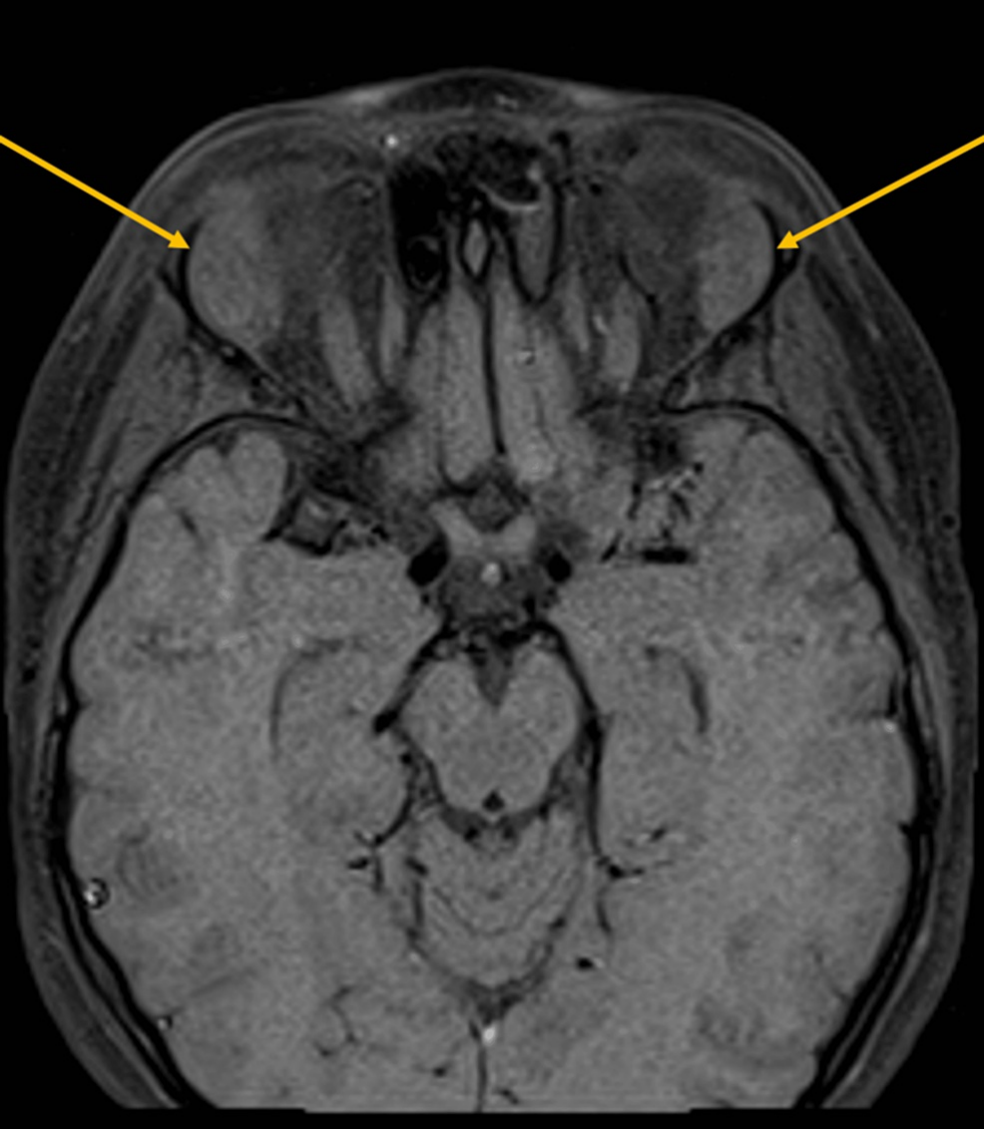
Axial MRI following gadolinium contrast demonstrating mild, homogenous contrast enhancement
